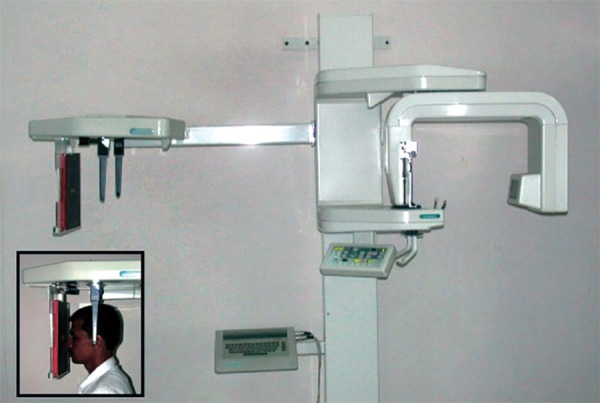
Planemecca PM 2002 CC Proline Panoramic X-ray unit (Inset-picture of standard patient position)
